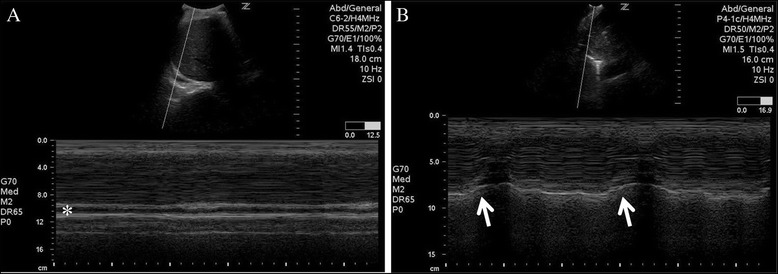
M-mode ultrasound images during respiratory cycles. The right image (a) demonstrated loss of respiratory movement of the right hemidiaphragm, while in the left image (b), normal respiratory movement of the left hemidiaphragm was detected (arrows inspiratory phase). A small pleural effusion (aechoic space) was seen posterior to the diaphragm (asterisk)
